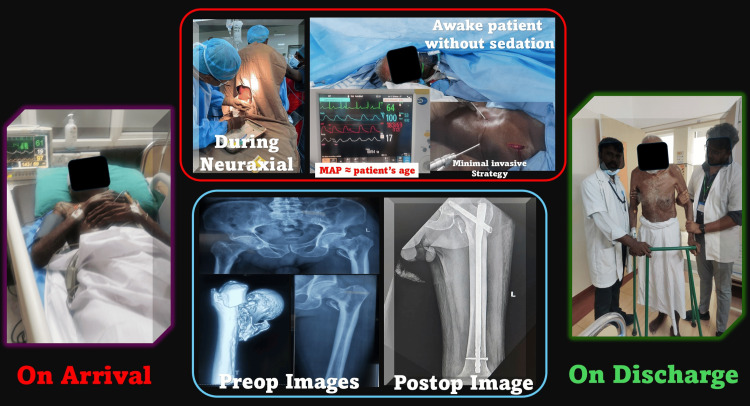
Perioperative course of a 111-year-old centenarian undergoing hip fracture fixation under regional anesthesia. The sequence of clinical images depicts the patient’s journey from admission to discharge. On arrival, the patient was monitored and stabilized before surgery. Intraoperative images show the administration of combined spinal-epidural anesthesia with stable hemodynamics, and the patient remained awake without sedation, maintained with a minimal invasive strategy. Preoperative radiographs confirmed an intertrochanteric femur fracture, and postoperative radiographs demonstrated successful fixation with intramedullary nailing. The final image shows the patient mobilized with support at discharge on postoperative day 7, highlighting functional recovery. MAP, mean arterial pressure; Preop, preoperative; Postop, postoperative Source: this figure was created by the first author, KS.
